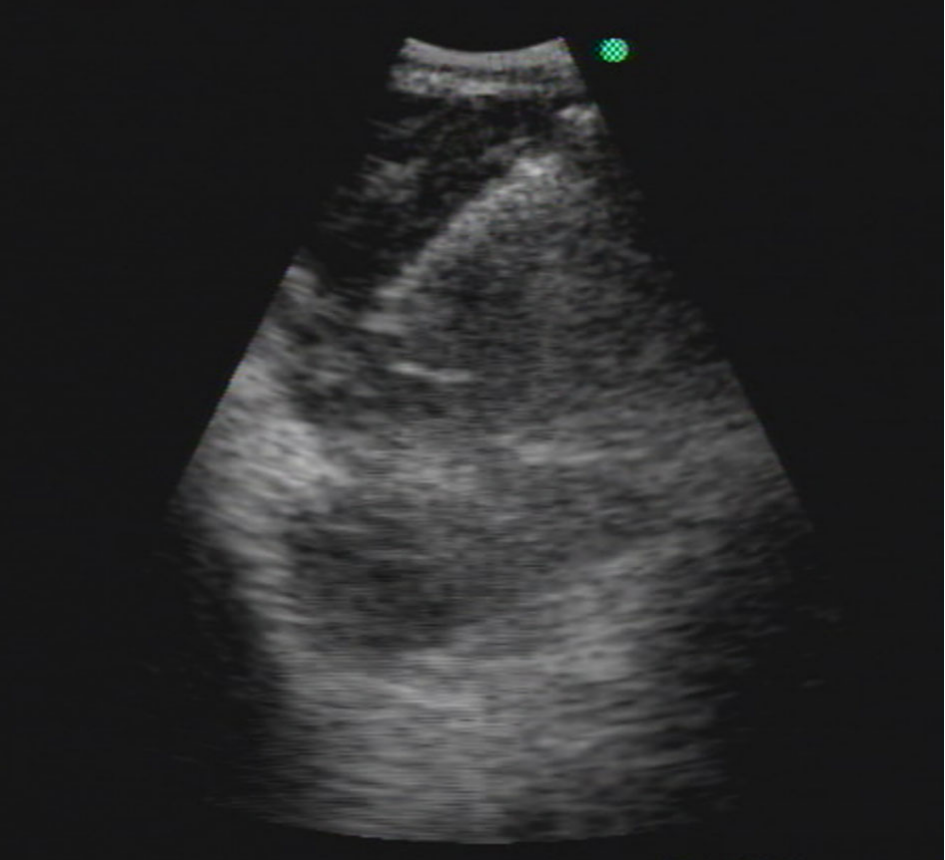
Endobronchial ultrasonography‐guided transbronchial needle aspiration (EBUS‐TBNA)
